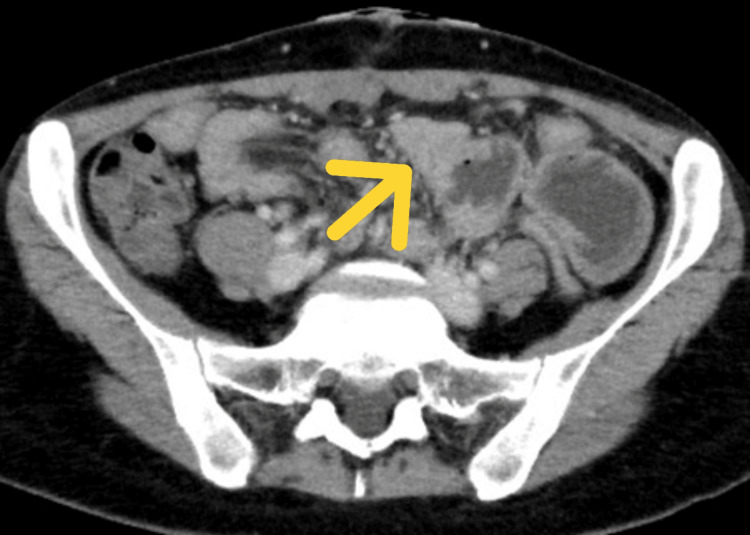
Axial contrast-enhanced CT revealing marked circumferential wall thickening of the upper jejunum The arrow indicates the upper jejunal wall thickening.
